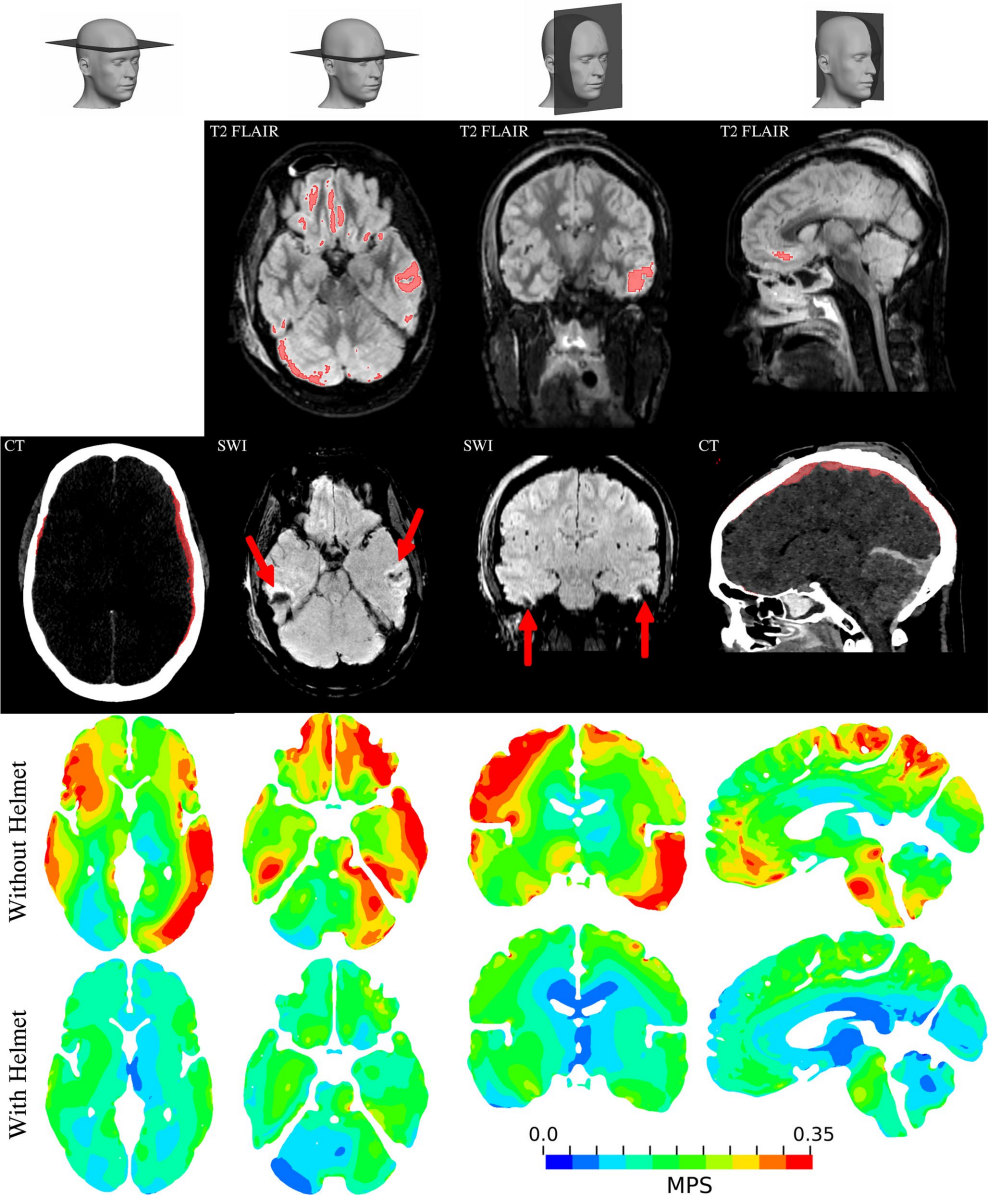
Lesions detected in the victim’s MRI and CT, along with corresponding sections of ADAPT model, showing the accumulated MPS distributions over the brain. The MPS are plotted for the reconstruction both with and without a helmet (without LFL)
